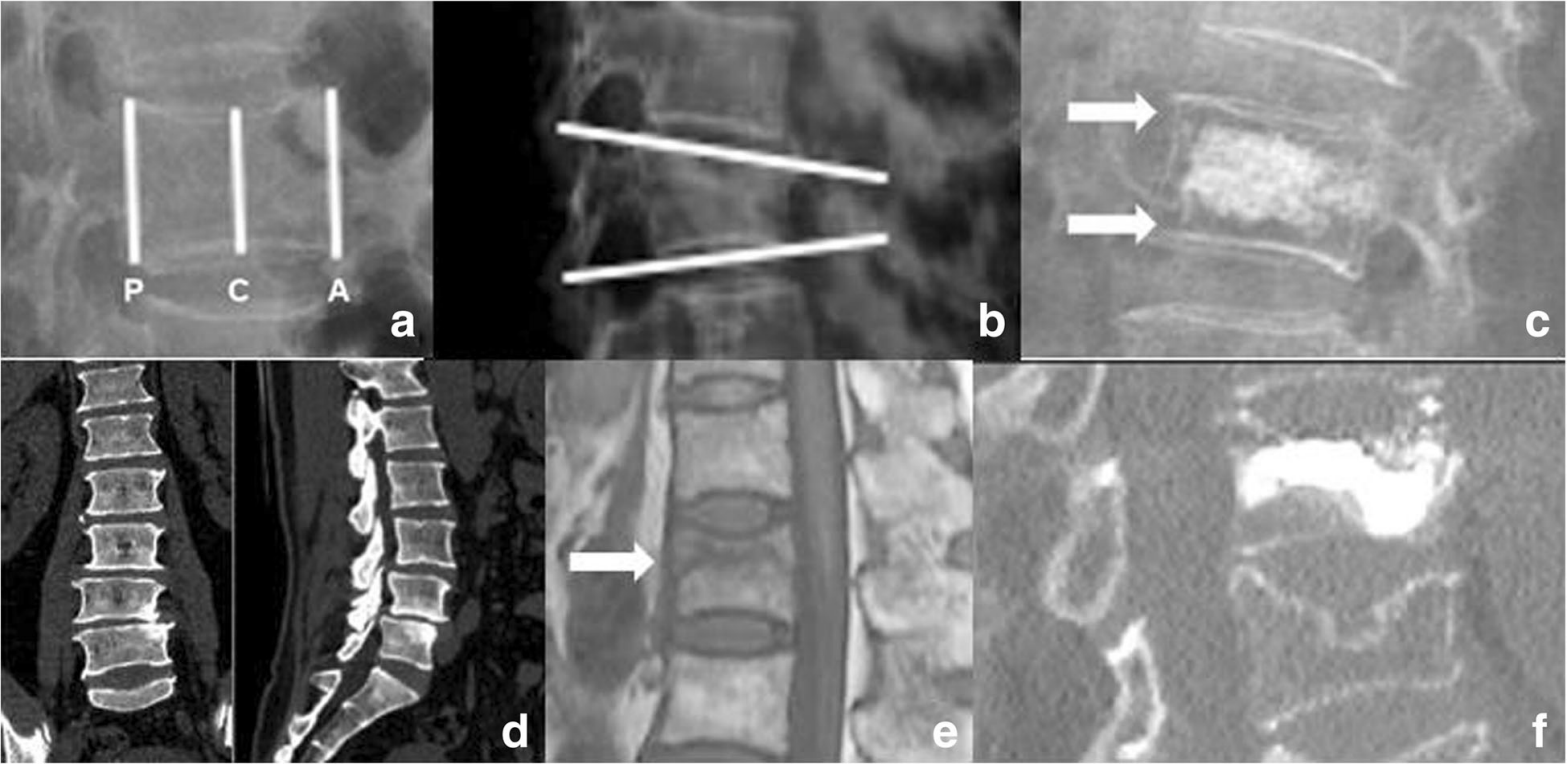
a (A): anterior vertebral height, (C): central vertebral height, (P): posterior vertebral height. b Kyphotic angle (KA). c Cement non-contact both endplates (NPEC). d CT images. e MRI image of OVCF with IVC. f Postoperative CT images, some part of the vertebra is not filled with cement
